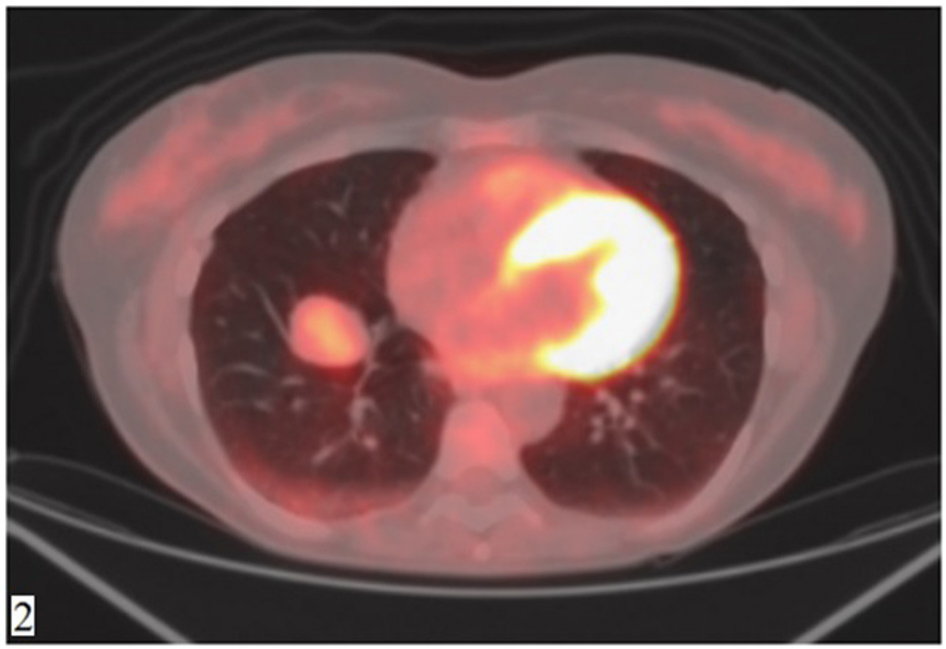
FDG-PET showing mild contrast uptake at the level of the nodule (SUV max 2.8).
